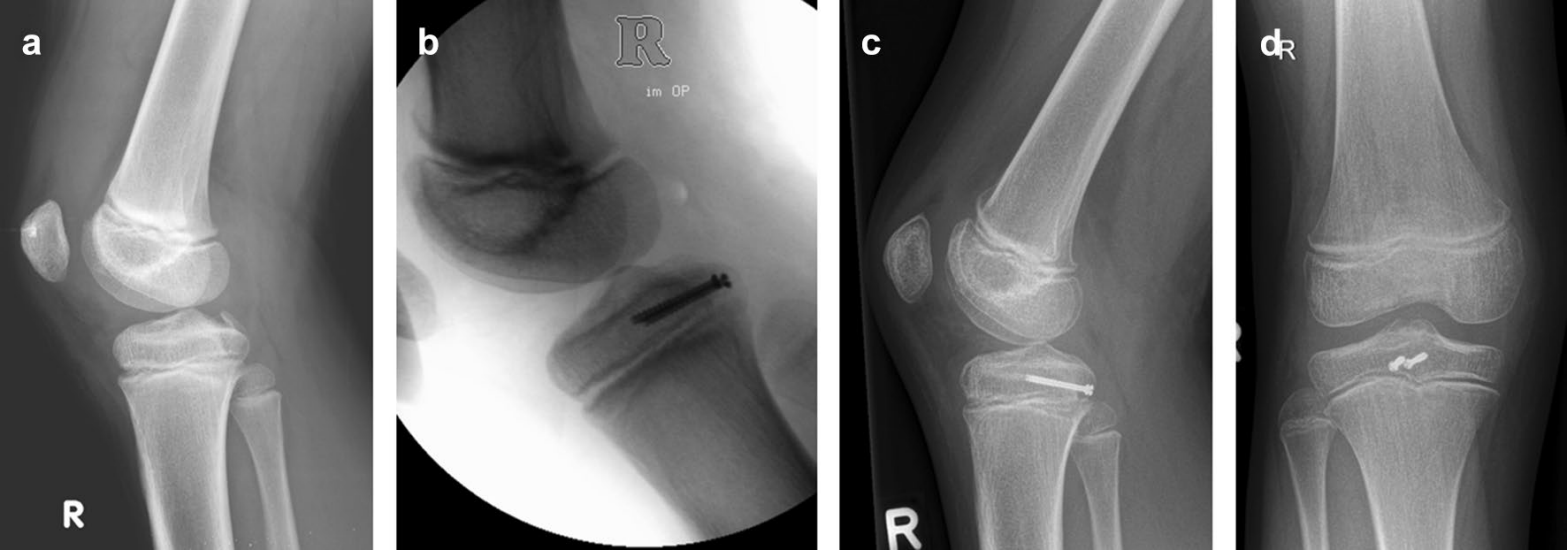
Example of a 10-year old patient with a displaced avulsion fracture of the PCL sustained in a road traffic accident (a). The injury was treated with open reduction and screw fixation (b). Postoperative radiographs showing anatomic reduction of the fracture (c, d)
